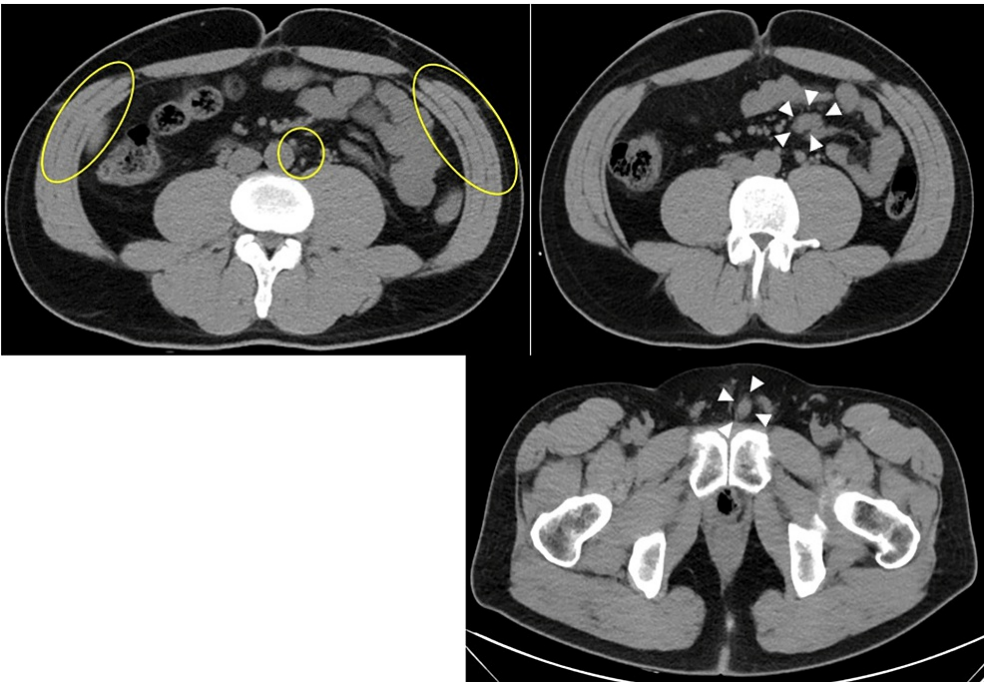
New lesions after the administration of enfortumab vedotin. Although computed tomography performed two months after the initiation of enfortumab vedotin (EV) exhibits metastases at other sites (white arrows), no re-growths of metastases that shrank with EV therapy were observed (yellow circles).
